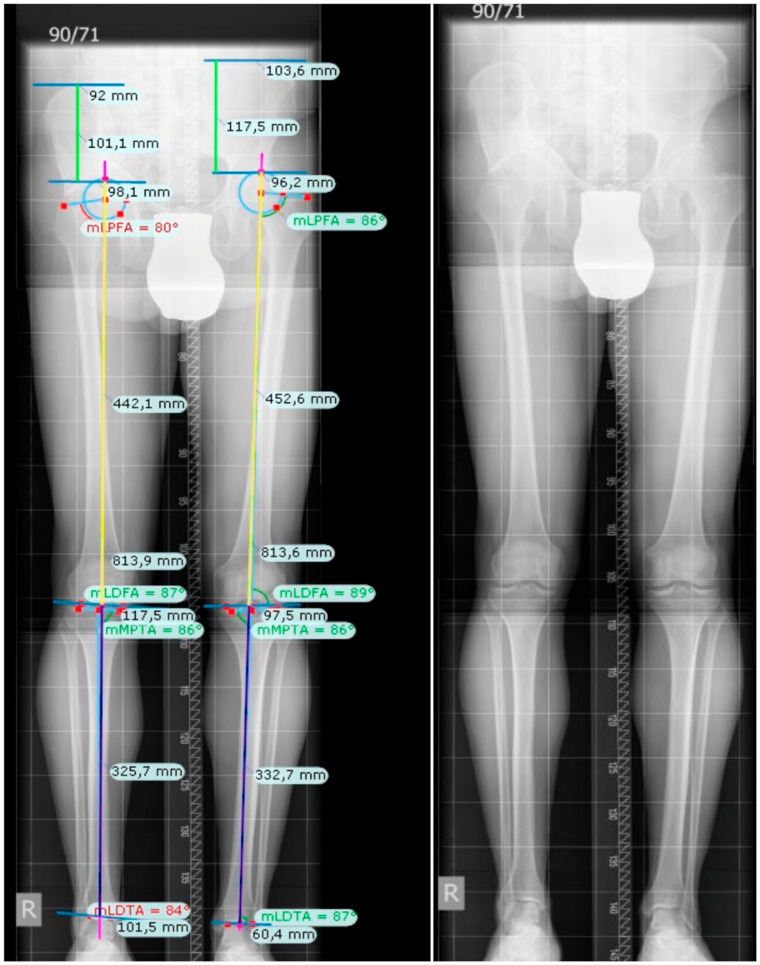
Exemplary radiograph with (left)/without (right) measurements for leg length difference and main angles; mLPFA mechanical lateral proximal femoral angle; mLDFA: mechanical lateral distal femoral angle; mMPTA: mechanical medial proximal tibial angle; mLDTA: mechanical lateral distal tibial angle.
